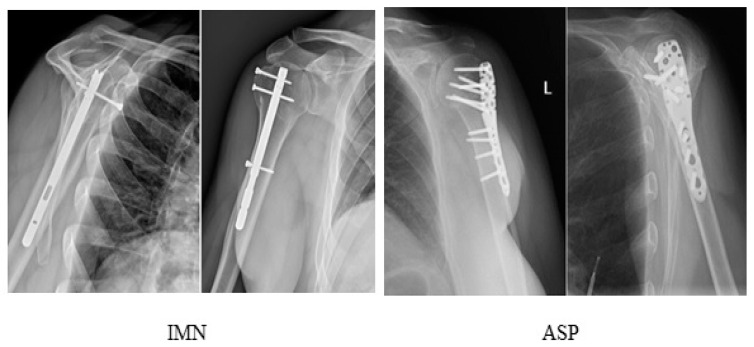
Radiographs of the shoulder at the 6th postoperative month after stabilization with IMN and ASP.
